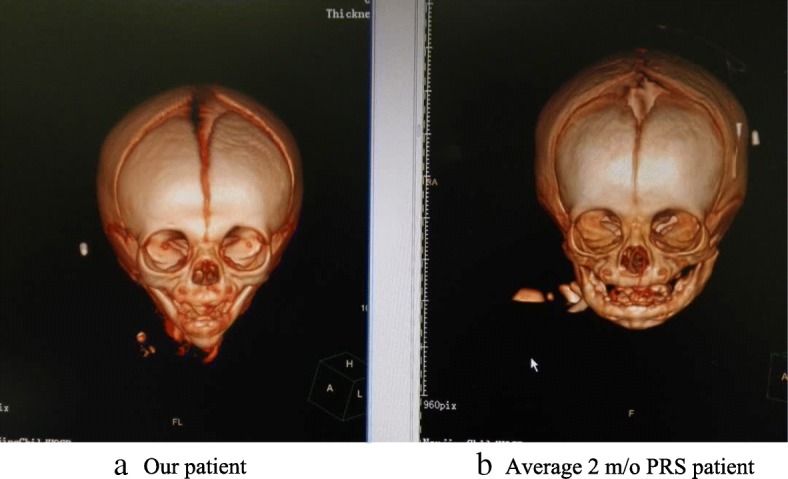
a shows the Craniofacial CT reconstruction of our patient. b shows the Craniofacial CT reconstruction of a normal 2-months-old PRS patient
